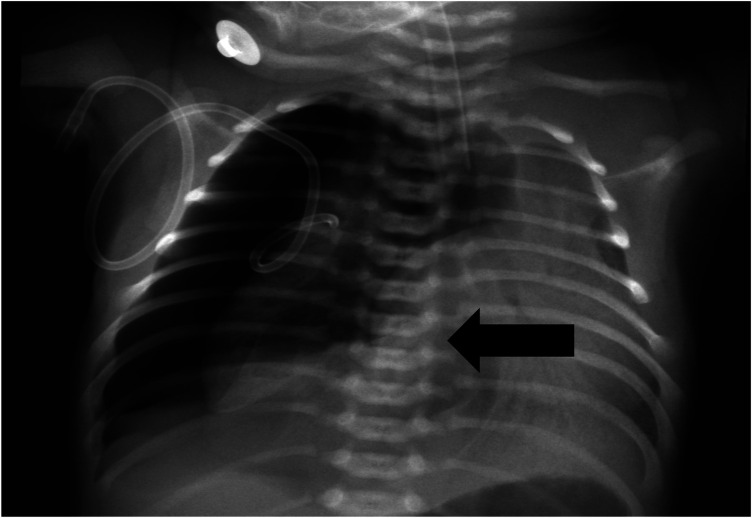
Chest x-ray showing right-sided pneumothorax with mediastinal shift.
